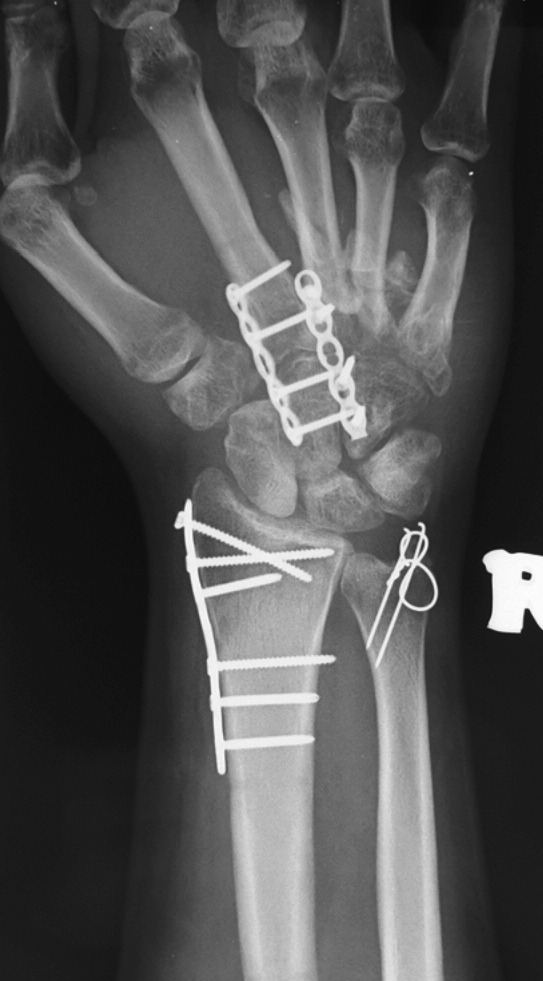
Radiograph shows the configuration of bones of patient's right hand after fixation.
